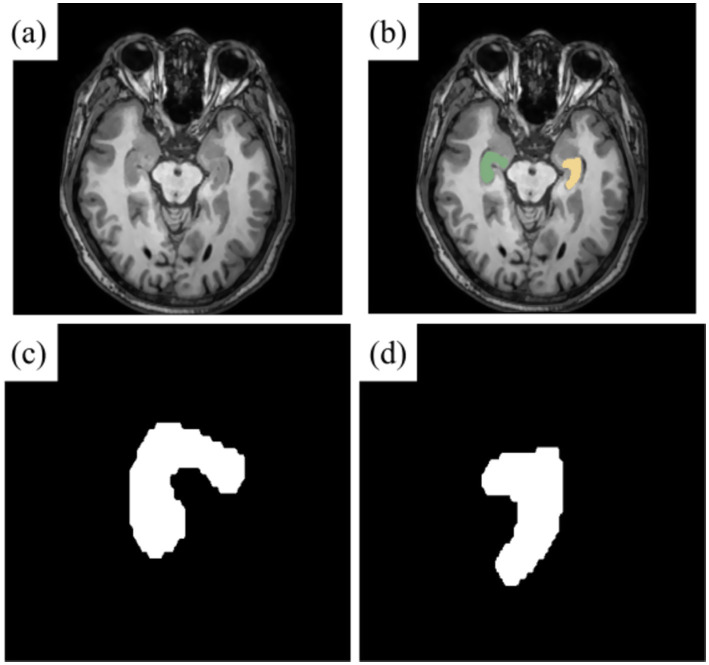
Example of hippocampus segmentation. (a) Original MR image, (b) Label sample, (c) right hippocampus segmentation map, (d) left hippocampus segmentation map.
